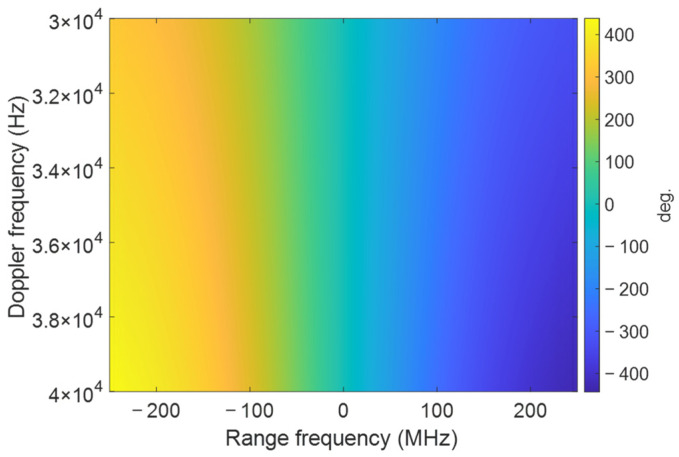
The compensation phase in the 2D frequency domain with a non-zero Doppler frequency. The range of $f_{\eta }$ is [30,000, 40,000] Hz.
